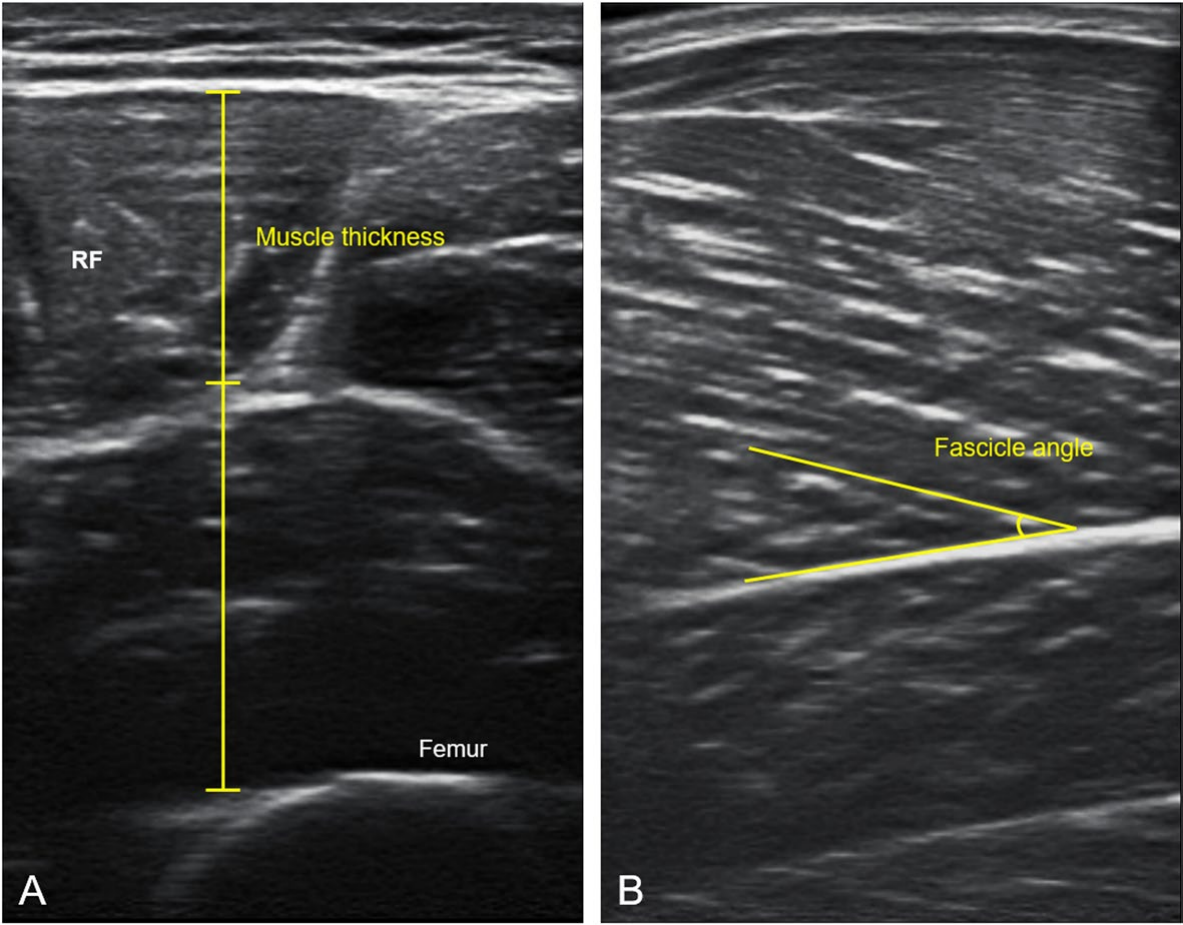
Representation of muscle architecture measurement of rectus femoris (RF) at the sonographic image (A) muscle thickness; (B) fascicle angle.
